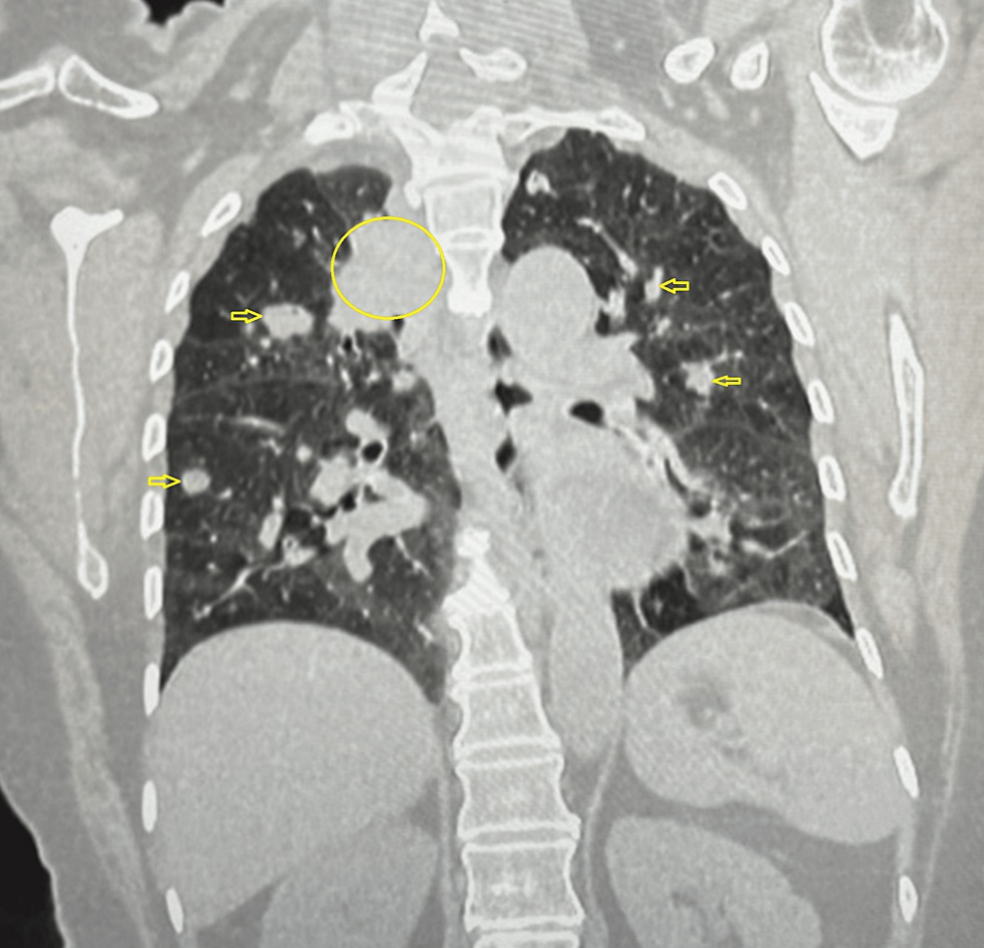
Contrast-enhanced computed tomography scan of the chest showing a large irregular apical mass lesion and multiple pulmonary metastases. Yellow circle: Irregularly shaped mass lesion in the apical segment of the right upper lobe measuring 33 x 30 x 40 mm in size. Yellow arrows: Multiple cavitary and non-cavitary pulmonary metastases.
